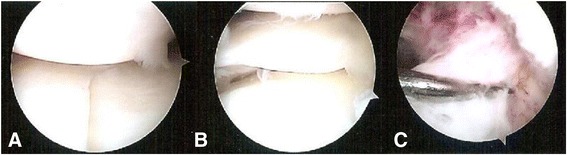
A second-look arthroscopic evaluation. They were compatible with postoperative plain radiographs. a A solid union of the fracture without a step-off over the medial and lateral tibial condyles. Good healing of the articular surface with fibrocartilage. b A well-healed lateral meniscus after arthroscopically assisted suture repair. c A strong and stable anterior cruciate ligament (ACL)
